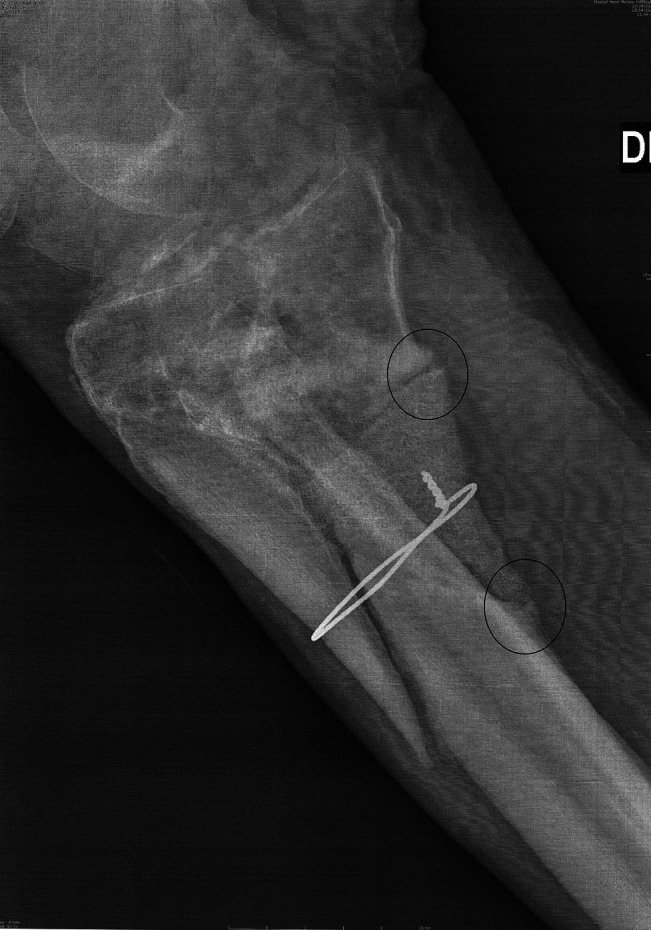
Radiograph of the right tibia performed 7 months after ceramic implantation and 4 months after external fixator removal showing a growth of bone in contact with the ceramic (circles)
